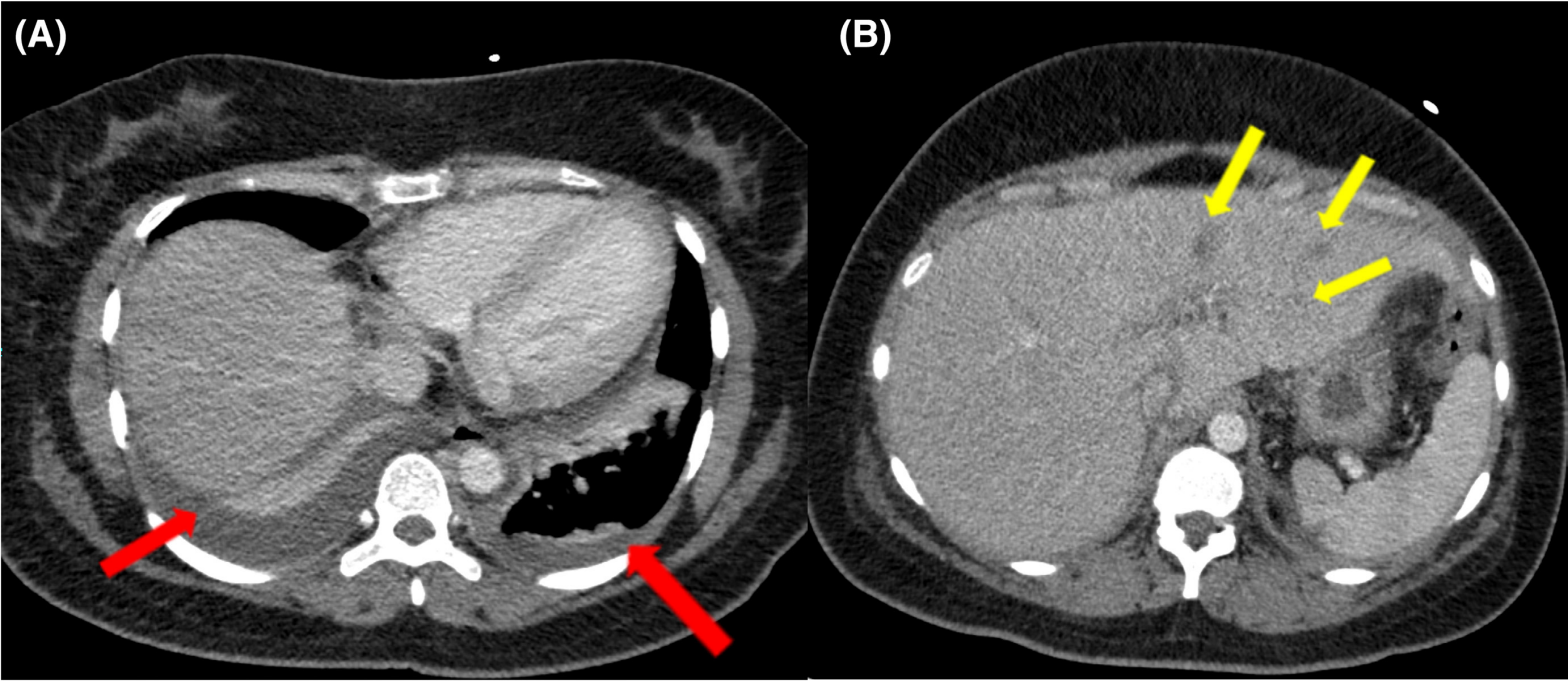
CT chest with intravenous contrast (A) Axial, mediastinal window cuts show bilateral pleural effusion (Red Arrows) with underlying collapse consolidation. (B) Axial, upper abdominal cut demonstrates multiple, small, ill‐defined hypodense foci in the left lobe of liver (Yellow arrows).
